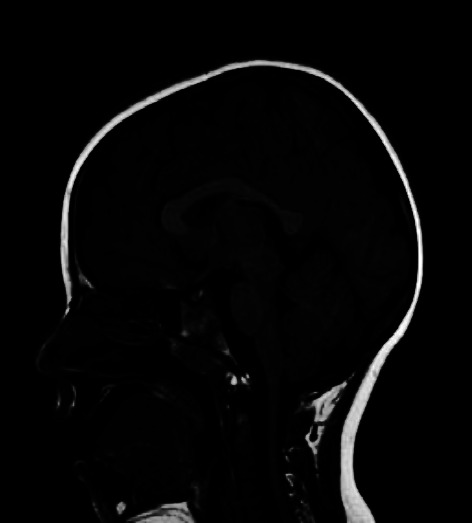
MRI sagittal seq. (5 yrs): P.2 showed a mild thickening of CC, an mild herniation of right cerebellar tonsil.
